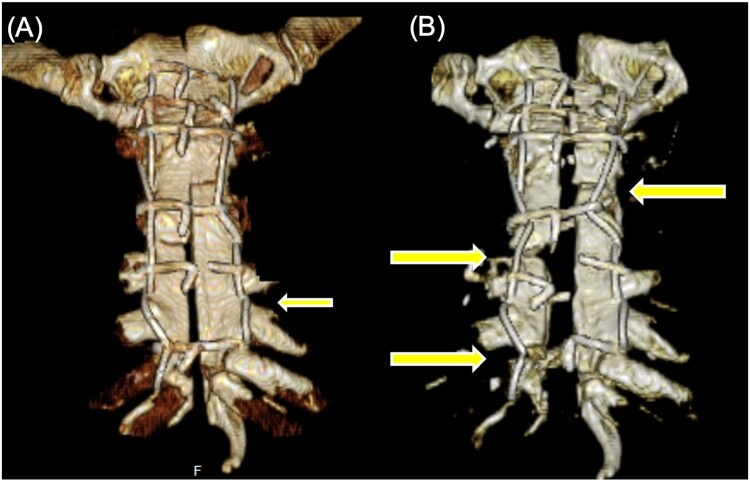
Serial 3 D-CT Images Showing Progression of Sternal Dehiscence. (A) Wire fracture (arrow) and lower sternal dehiscence after initial closure. (B) Progressive sternal fractures (arrows) and worsening dehiscence prior to reoperation.
